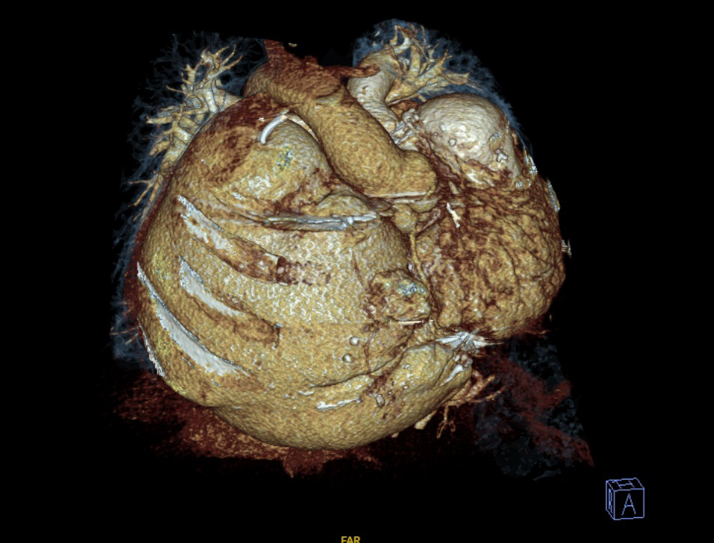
Cardiac Computed Tomography Three-Dimensional Reconstruction Showing a Markedly Dilated Right Atrium
